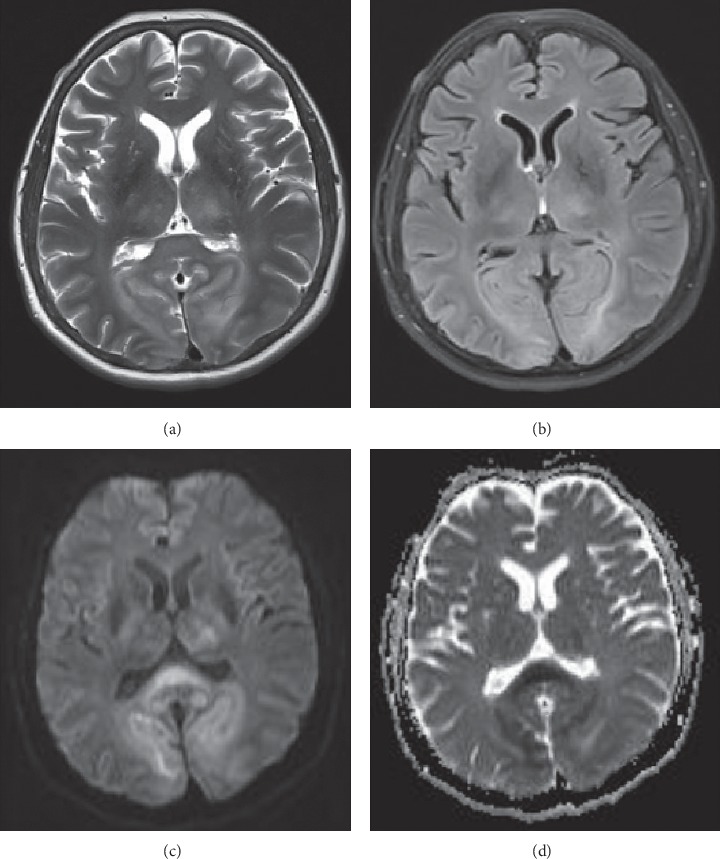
Brain MR (axial images) obtained 2 days after angiography. T2 and FLAIR images (a, b) show bilateral symmetrical hyperintensities in basal ganglia, in parieto-occipital cortex, and in splenium of corpus callosum. DWI and ADC images (c, d) demonstrate restricted diffusion in the same areas.
